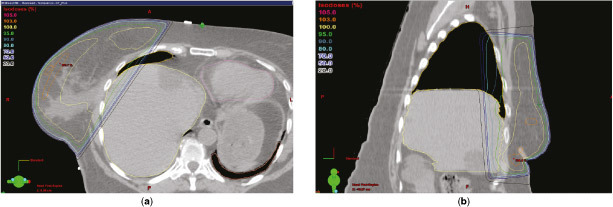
Free-breathing transverse (a) and sagittal (b) planning CT images from Eclipse™ planning system (Varian Medical Systems, Palo Alto, CA). The delineated liver (light yellow contour line), right lung (dark yellow contour line), left lung (orange contour line) and heart (pink contour line) are shown in relation to the target field. A large volume of liver is within the target field, including in the high 95% isodose region (bright green contour line).
